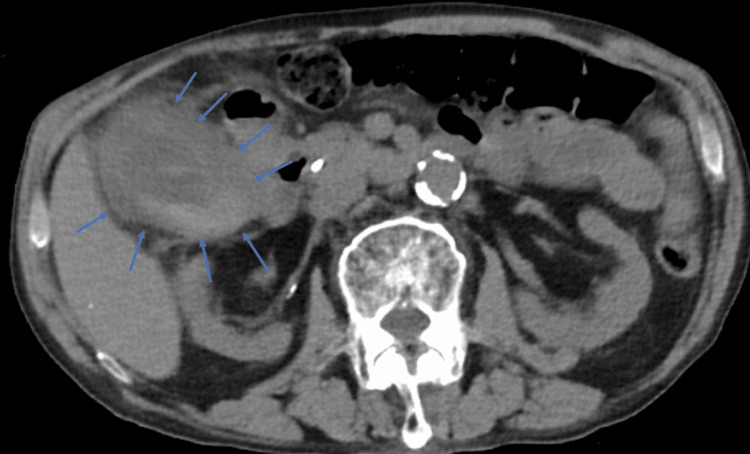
Abdominal computed tomography image showing gallbladder wall thickening and increased pericholecystic fat density. The blue arrow indicates the gallbladder wall.
